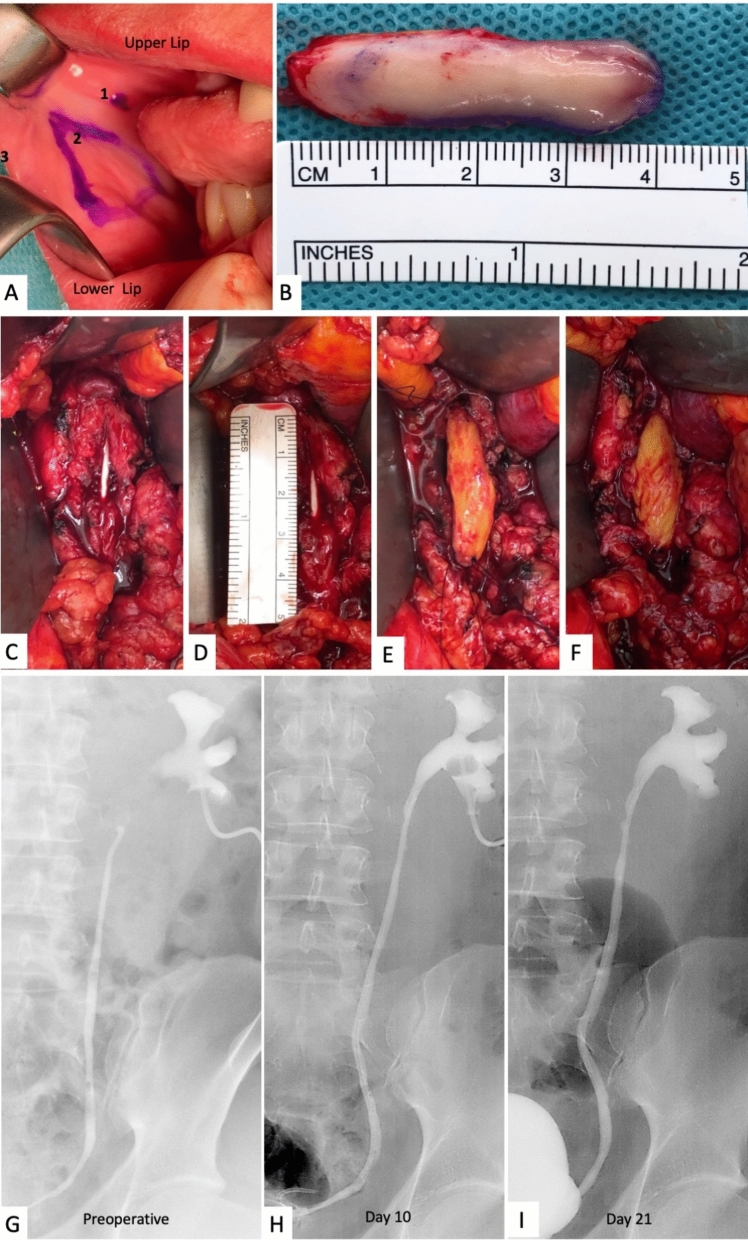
A: BMG donor site with “1” parotid duct, “2” buccal mucosa graft (BMG) marked with a pen, and “3” oral commissure. B: BMG after harvesting. C Lengthwise incised ureteral stricture with double-J stent placed in the ureter. D Measurement of defect. E onlay buccal mucosa graft (BMG) with sutures placed at the cranial and caudal commissure, and F showing the final placement of the BMG with finished continuous sutures along each side. G–I show a series of X-ray urographies of one patient, preoperative, 10 days postoperative and 21 days postoperative, respectively
